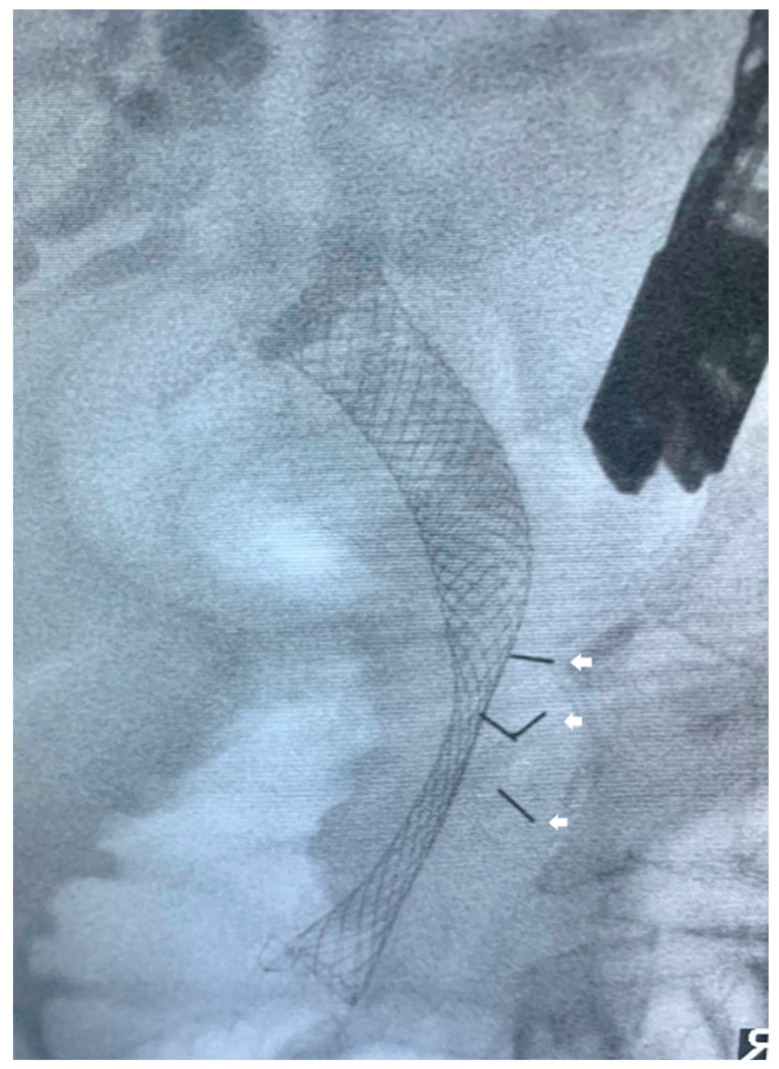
Metal stent in the bile duct with endoscopic ultrasound-guided Visicoil^TM^ fiducials (white arrow) placement in a patient with pancreatic head cancer for stereotactic body radiotherapy.
